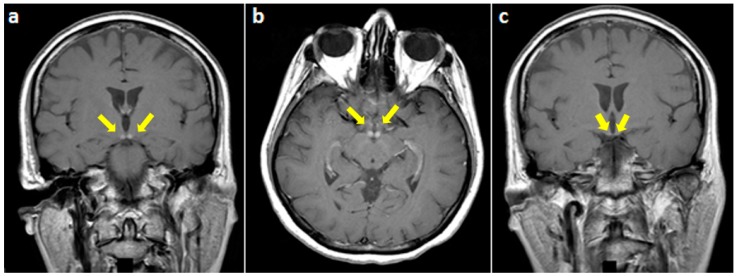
T1-weighted gadolinium-enhanced MRI at the level of mammillary bodies (yellow arrows) ((a) coronal and (b) axial views) and (c) the third ventricle showing an isolated enhancement of the former.
